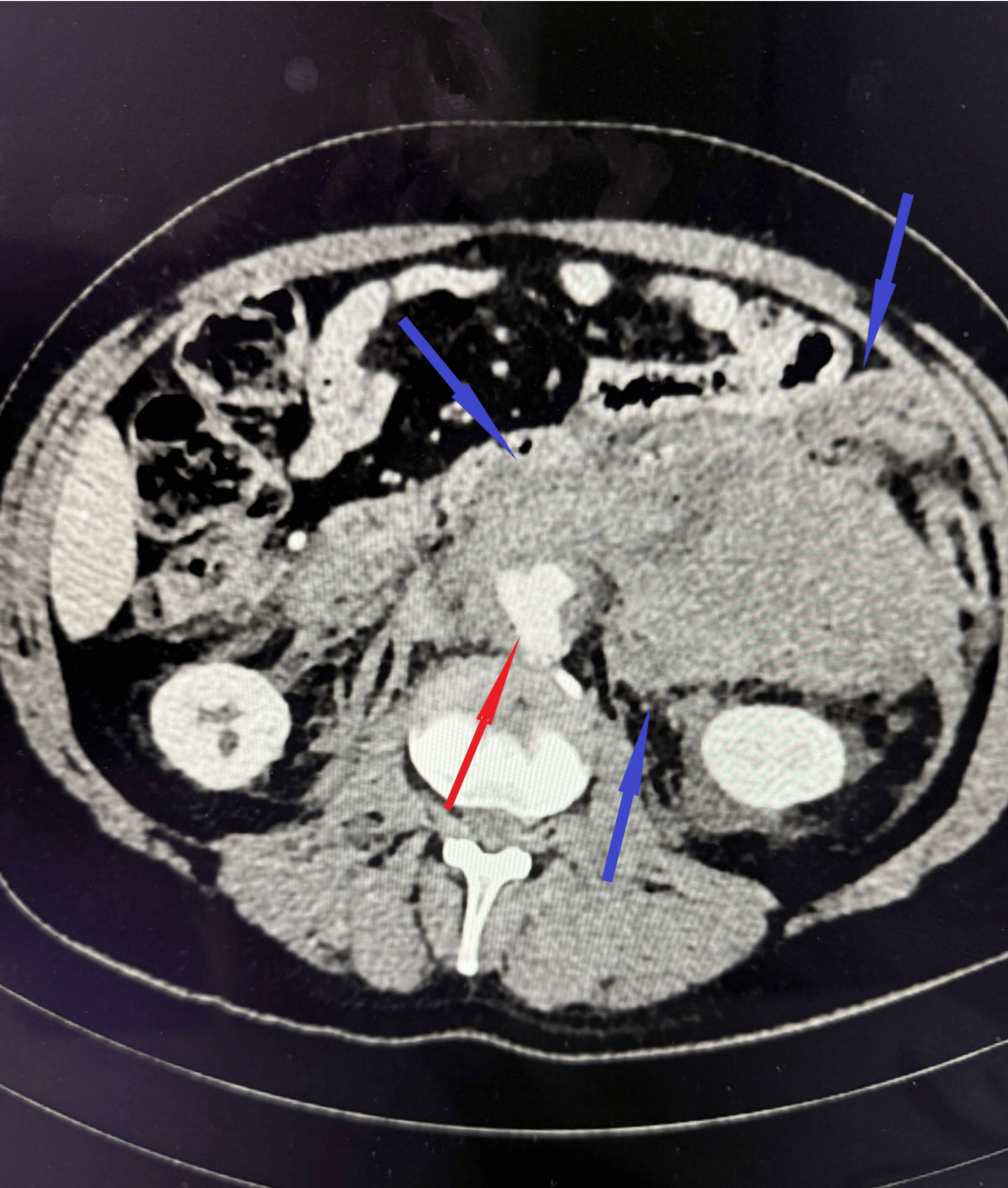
Contrast-enhanced CT Contrast-enhanced CT demonstrating a distal abdominal aortic aneurysm (red arrow) at the level of the aortic bifurcation, measuring approximately 40 × 40 mm with a length of ~35 mm, irregular contours, and an associated mural thrombus (7-8 mm). Extensive bilateral para-aortic retroperitoneal hemorrhage, more pronounced on the left, is present, forming a large organized hematoma (blue arrows) measuring 8.7 × 15.2 × 25.3 cm.
